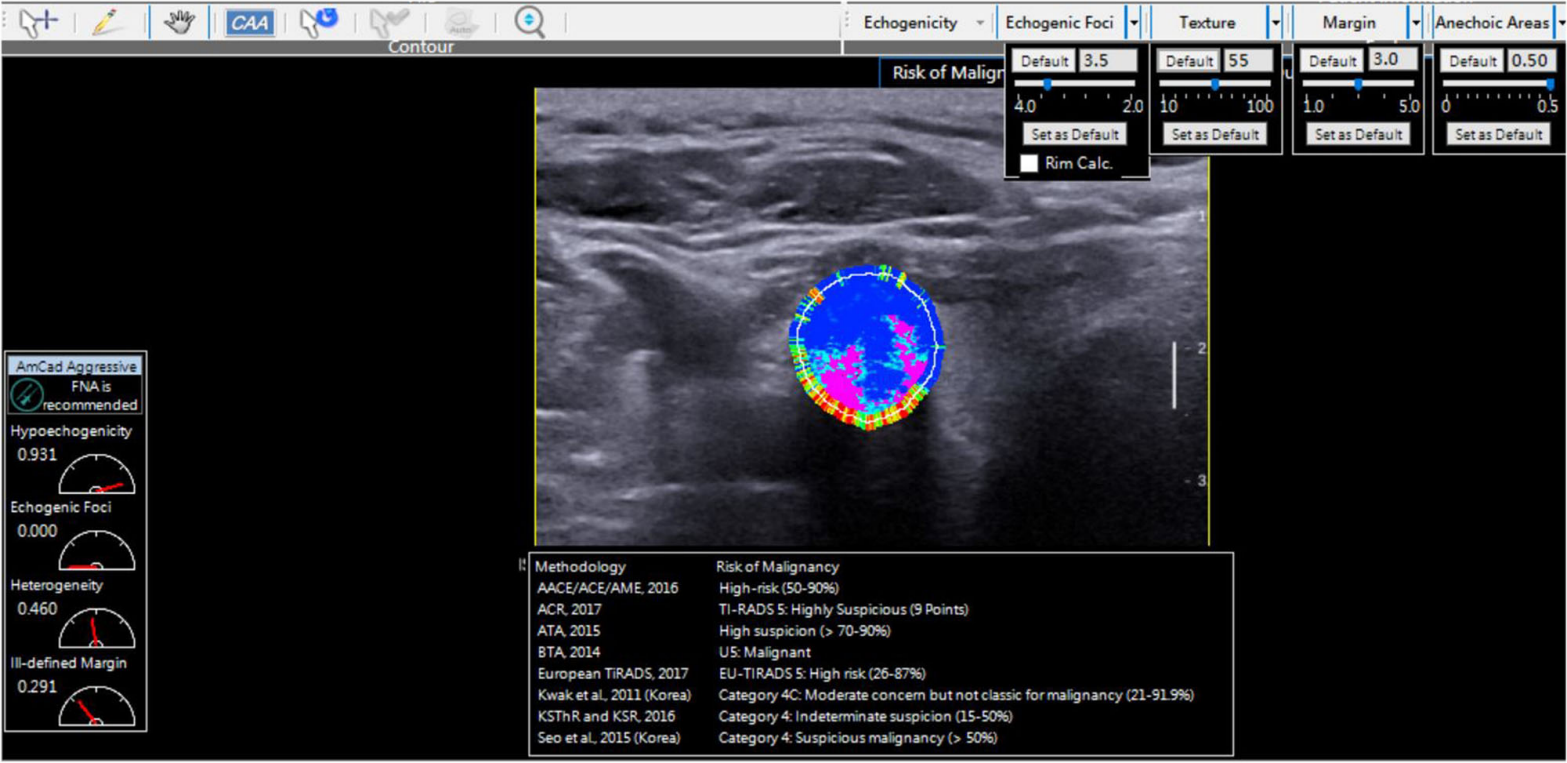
Interactive interface of the AmCAD diagnosis system, demonstrating its automated identification and precise quantification of ultrasound attributes, while also providing an accurate assessment of TI-RADS grade in thyroid nodule
